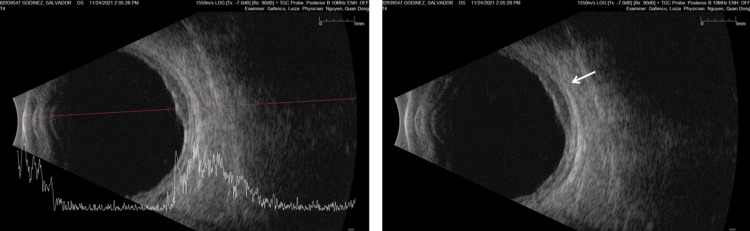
B-scan ultrasonography of the left eye (2021 visit) B-scan ultrasonography of the left eye demonstrating diffuse choroidal thickening measuring approximately 2.8–3.2 mm with medium-to-high internal reflectivity. No overt scleral involvement, extrascleral extension, or vitreous opacities were identified at presentation. The quantitative thickness measurement improves comparison with similar reported cases of choroidal lymphoma. OD, oculus dexter (right eye); OS, oculus sinister (left eye)
